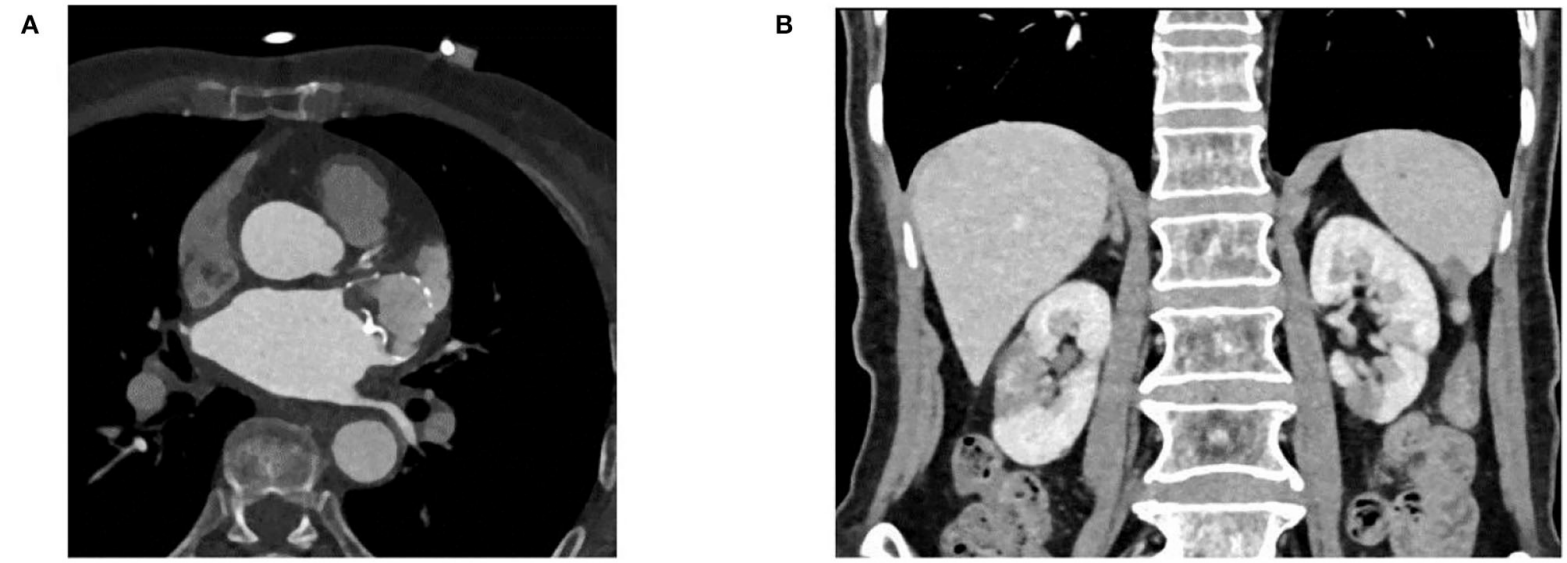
(A) Coronary artery CT angiography showing no protruding device-related thrombus and peri-device leak. (B) Renal artery CT angiography showing no new onset infarction.
